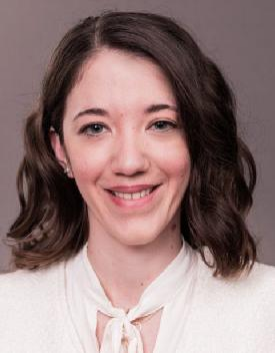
Giorgia Benzoni is a cardiology resident at University of Milan-Bicocca, Italy. During her residency, she developed a strong interest in cardiovascular imaging, with a particular focus on echocardiography and valvular heart disease. She is currently pursuing a fellowship in this field at Ramón y Cajal University Hospital in Madrid, Spain. In addition to her clinical training, she is involved in research projects related to cardiovascular imaging and valvular heart disease.
